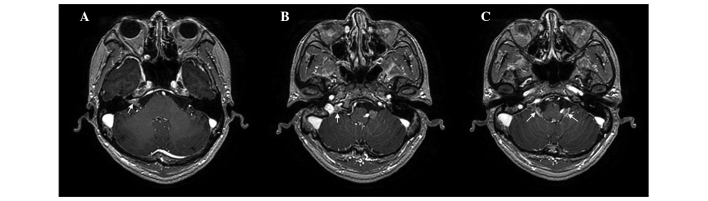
Magnetic resonance imaging of the brain showed: (A) Irregular nodular enhancement within the right internal auditory canal (arrow); (B) an enhancing lesion in the right jugular fossa (arrow); and (C) an enhancing mass in the bilateral cerebellomedullary cisterns (arrows).
